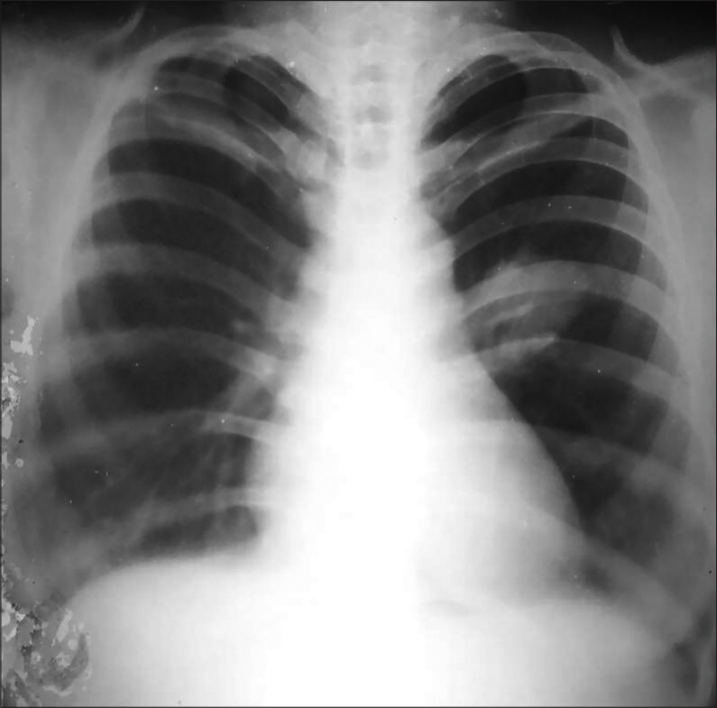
Chest radiograph shows a rounded mass lesion in the left
